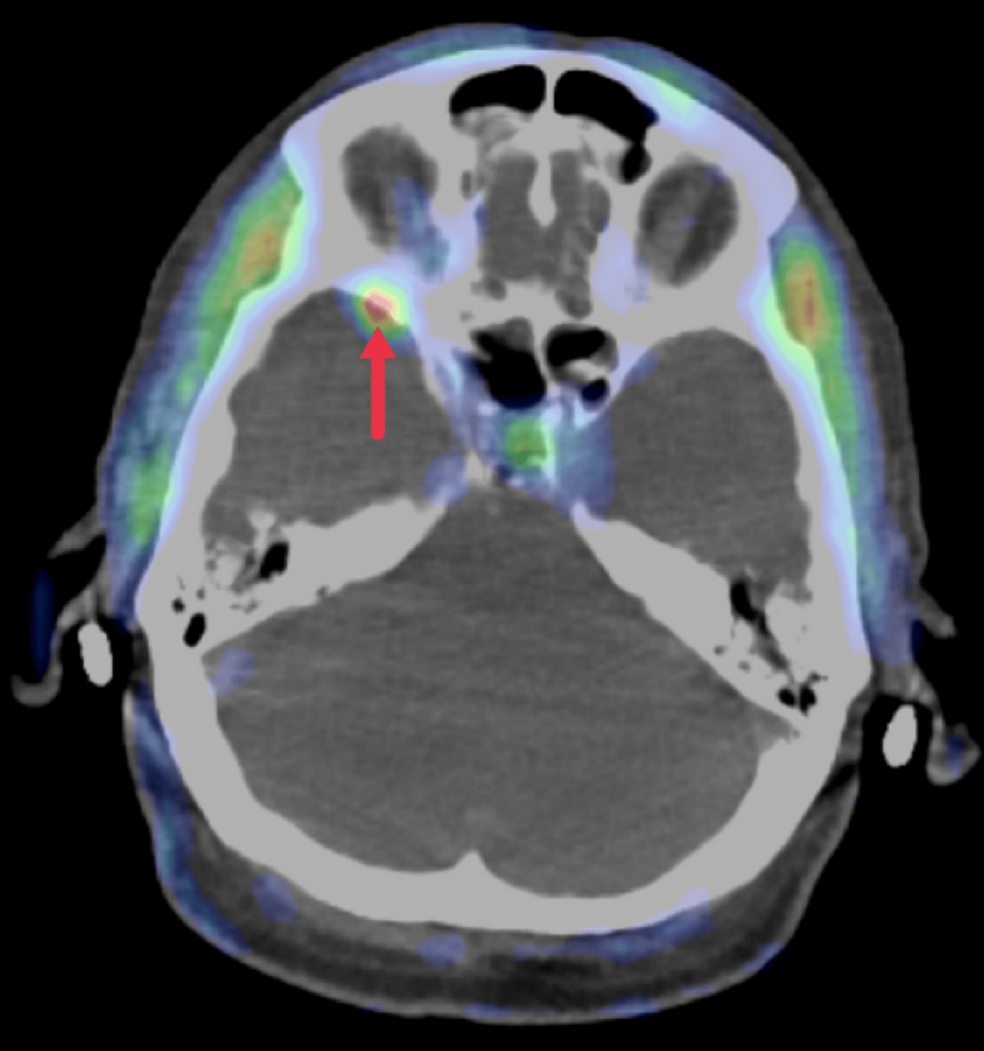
Axial 18F-fluciclovine PET/CT performed one year prior demonstrates increased uptake in the location of the described dural-based right middle cranial mass PET/CT: positron emission tomography/computed tomography
